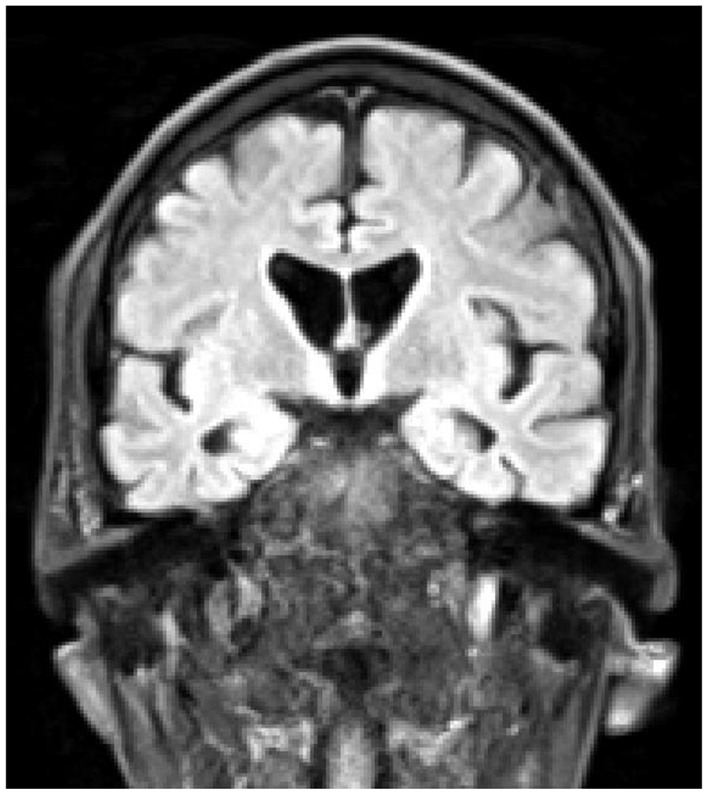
Coronal T1-weighted MRI scan, demonstrating atrophy of the caudate nuclei, and enlargement of the lateral ventricles.
